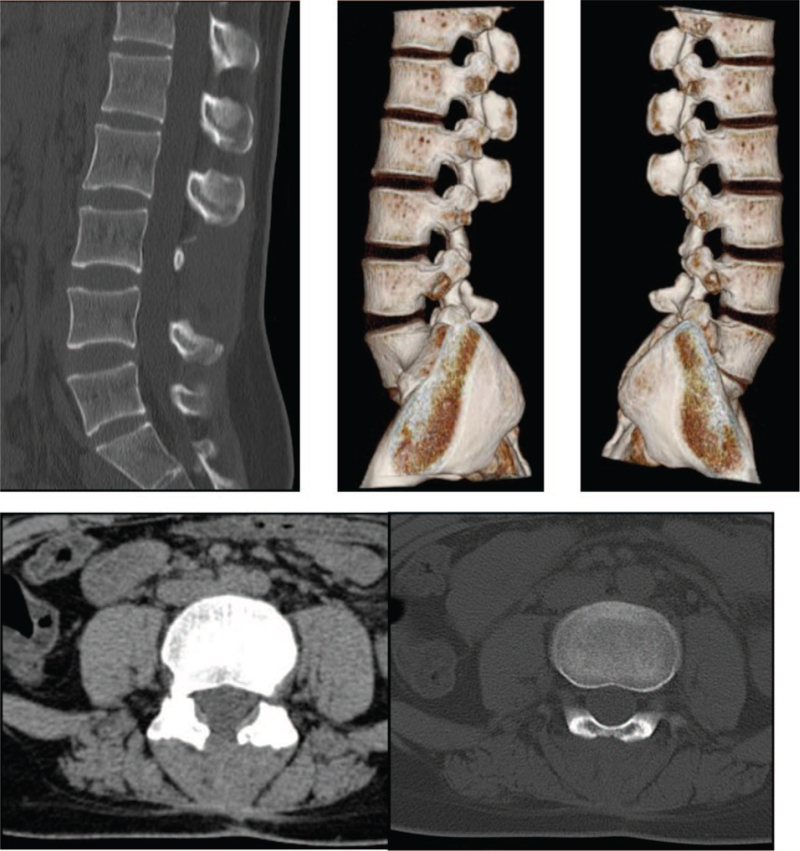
Preoperative MRI images & Postoperative MRI images. Preoperative: The normal bone in the right part of the L2 vertebral body, adjacent pedicle, and L3 accessory disappeared, and was partially replaced by abnormal mass-like and mass-like signals, such as slightly longer T1 and slightly longer T2. The lesion range of the L3 spinous process area was approximately 3.2 × 2.2 × 3.1 cm. Postoperative: The postoperative state of the lumbar spine showed absence of the L3 spinous process, good sequence, and curvature of the lumbar spine. The signals of the vertebral bodies and intervertebral discs are normal.
